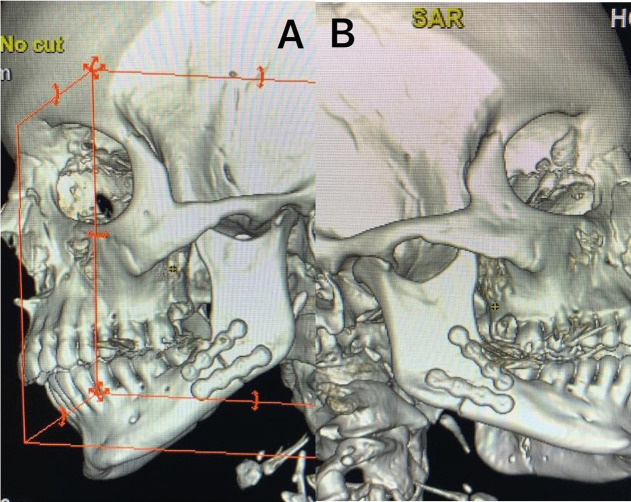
Postoperative computed tomogram demonstrating reduction and fixation fracture with good bone alinement. A: Left side. B: Right side.
